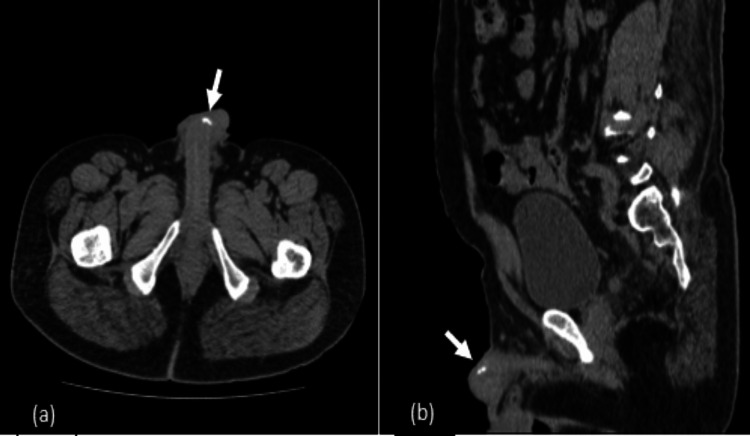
(a) Axial and (b) Sagittal CT images showing calcific plaque along the distal aspect of the shaft of the penis
